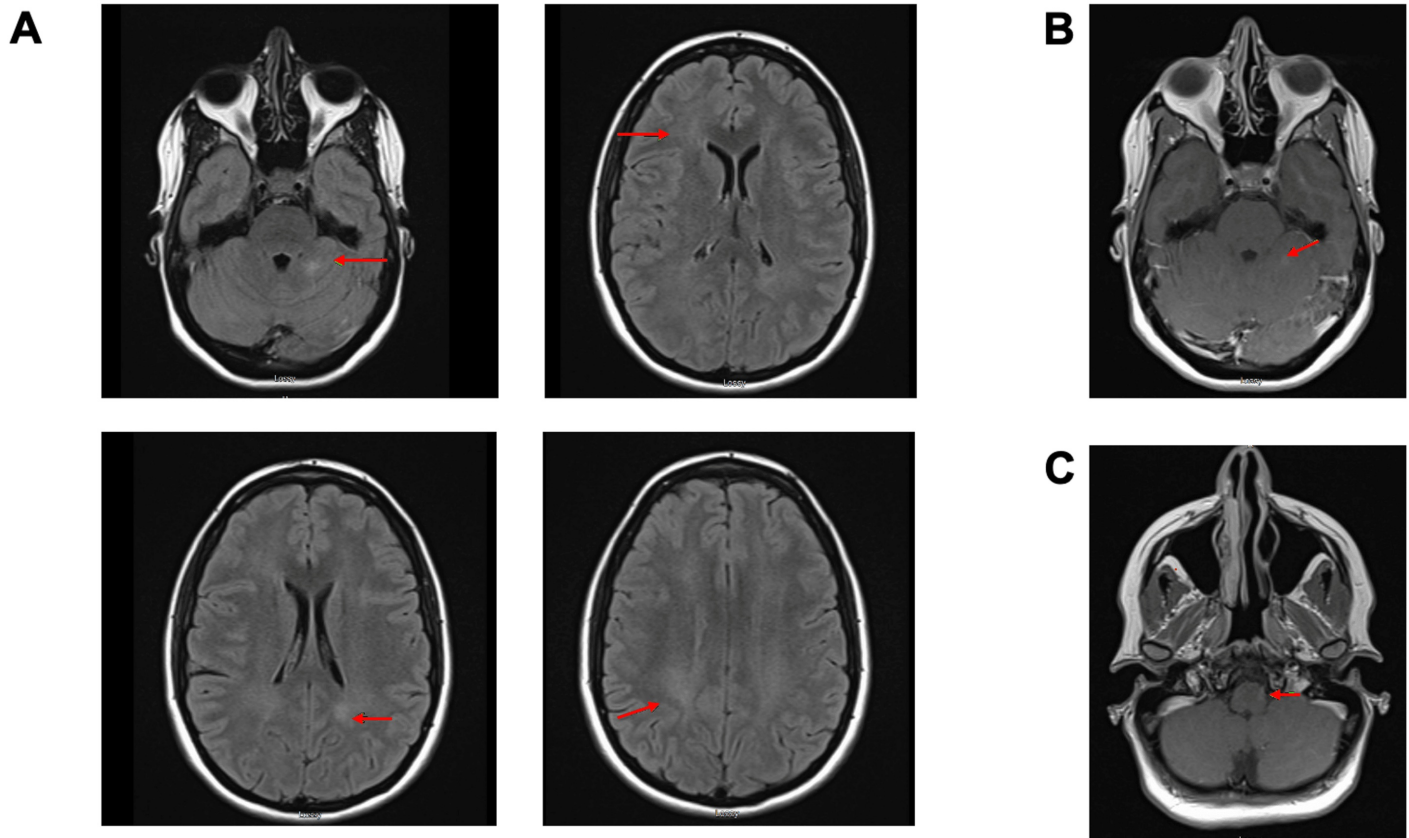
MRI brain (A) Non-enhancing fluid-attenuated inversion recovery (FLAIR) signal abnormality within the deep white matter of cerebral hemispheres marked by red arrows. (B) T1-weighted small enhancing lesion within left superior cerebellum marked by the red arrow. (C) T1-weighted linear enhancement along the left anterolateral surface of the medulla.
